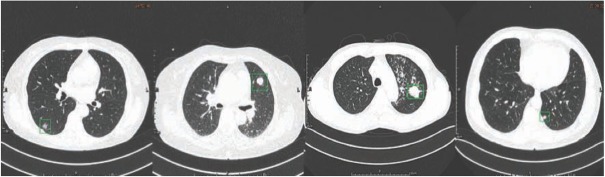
CT images of lung cancer pathological types: from left to right are ISA (adenocarcinoma in situ), SCLC (small cell lung cancer), SCC (squamous cell cancer) and IA (invasive adenocarcinoma). The green box areas are ROI areas of tumors.
